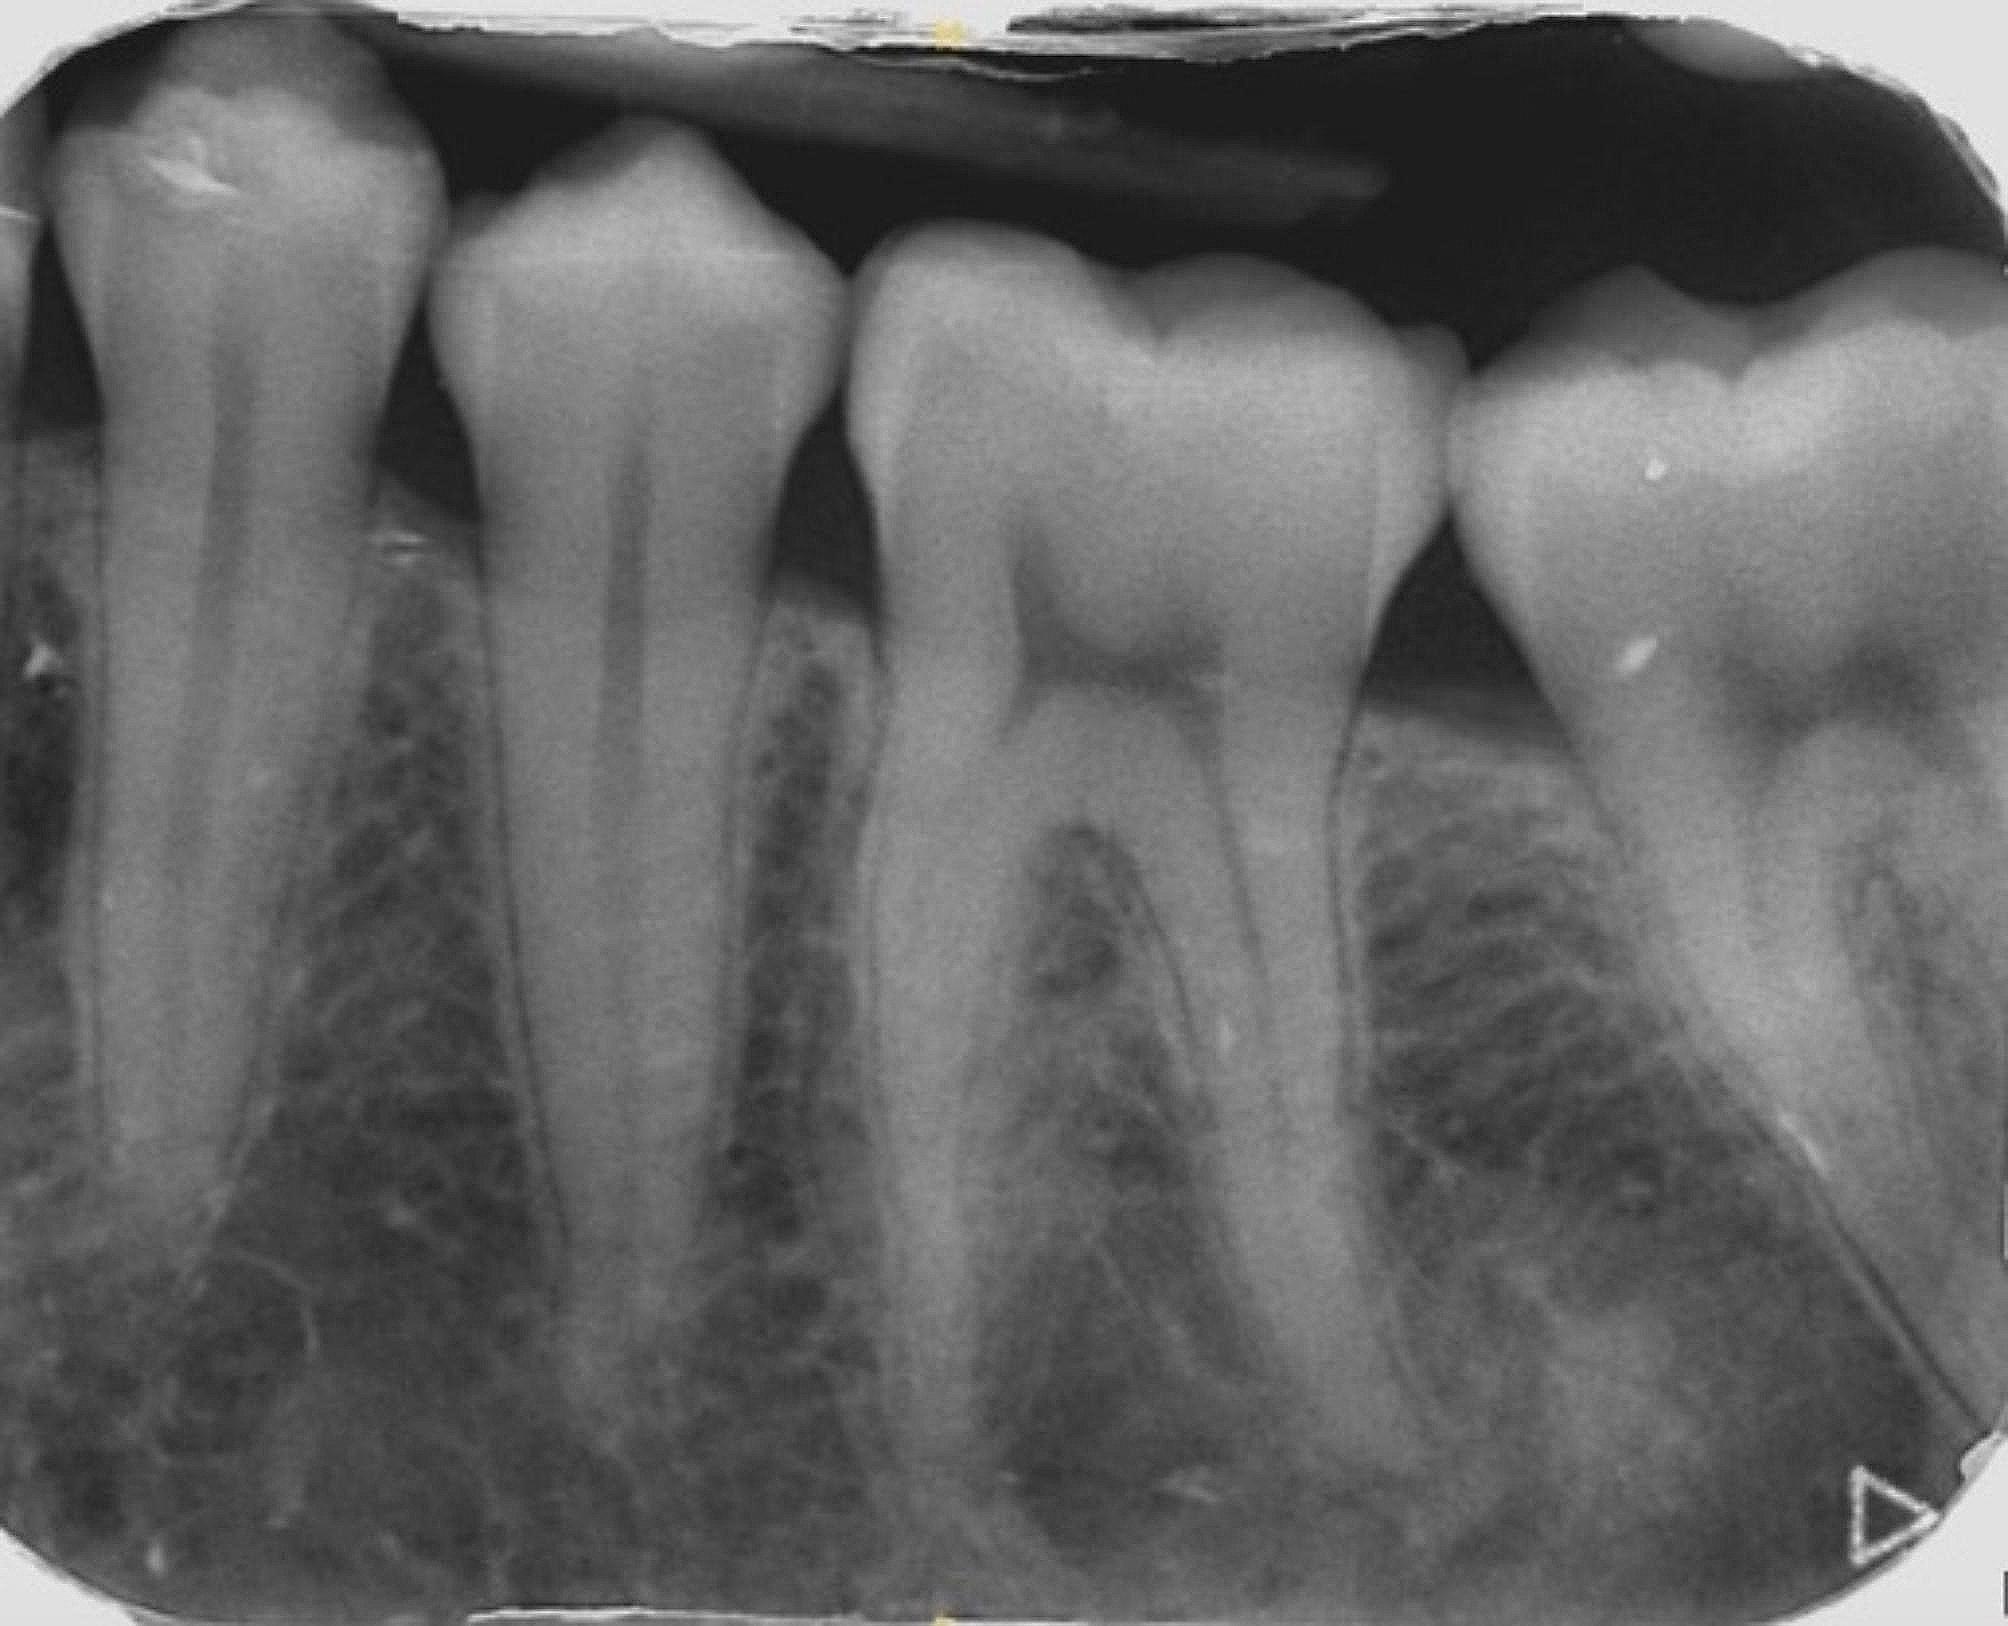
Retroalveolar X-ray of tooth #36 at 2 years of follow-up. Legend: This view’s showing sustainble complete reossification of the bony defect around root of tooth #36. A slight resorption a mesial root is also observed
